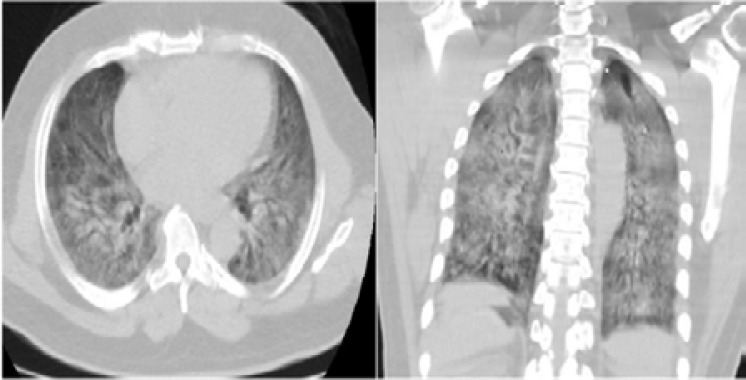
Axial and coronal reformatted unenhanced chest CT scan of a 63-year -old man with positive RT-PCR test and 7-days history of fever, cough and dyspnea showing consolidation with air bronchograms in bilateral posterior basal distribution; typical features of COVID-19 pneumonia.
